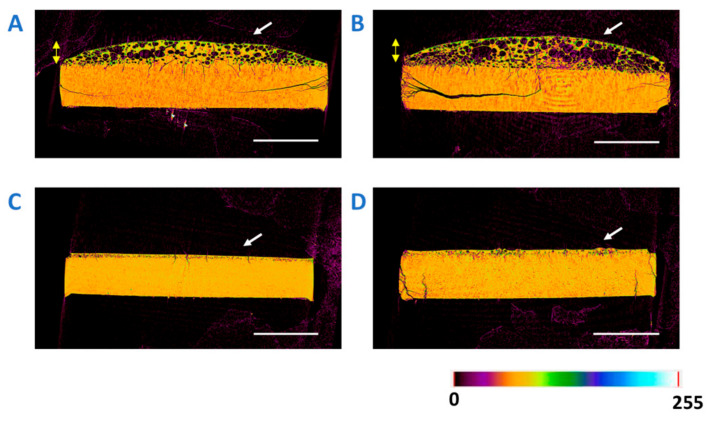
X-ray microcomputed tomography cross-sectional images for the 30% DL ASD tablets with 0% TPGS (A), 2% TPGS (B), 5% TPGS (C), and 10% TPGS (D) after 10 min of dissolution. Scale bar (in white) is 2 mm. Arrow (in white) on the images is pointing toward the dissolution front of the tablet. The color bar represents the range of density measurement from lowest (0) to highest (255).
